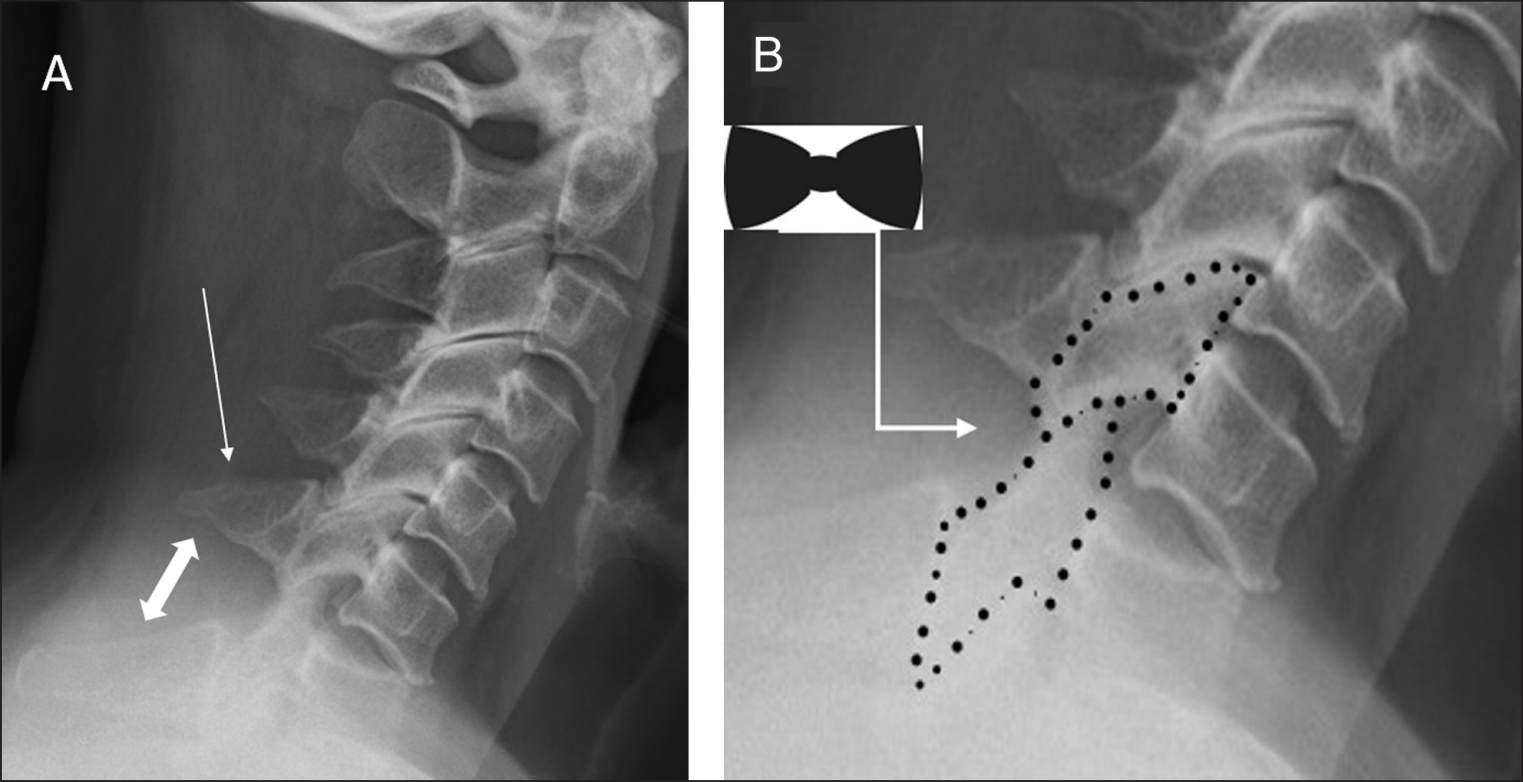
Bow-tie sign and laminar space sign. Lateral radiograph of the cervical spine (A) of a 33-year-old man following a fall from an electric scooter shows a grade I anterolisthesis with less than 25% anterior translation of C6 on C7 vertebrae. In addition, there is an oblique fracture across the spinous process of C6 vertebra, which is consistent with clay-shoveler fracture (single-headed arrow). Besides focal kyphosis and reduced overlap of the articular processes, there is fanning or widening of the interspinous distance (double-headed arrow). The laminar space (which is the distance between the spinolaminar line and the posterior surface of the articular pillars) widens progressively from C3 to C6 level. It changes abruptly and narrows at the C7 level (B). These changes indicate a sudden rotational injury at C6–7 level. Displacement of the interfacetal joint with rotational deformity at this level produces a “bow-tie” appearance and a “double-appearance” of articular facets above the C6–7 level.
